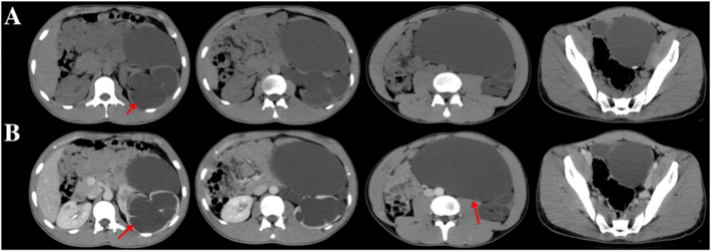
Case 5. CT scans obtained in a 20-year-old man with left congenital megaloureter. Nonenhanced scan obtained at the abdomen & pelvis shows huge fluid-filled loops (*red arrow*), and the normal bowel loops are compressed to the right side of the abdominal wall (a). The left renal pelvis and calyces show marked dilation, with parenchyma thinning (*red arrow*) on parenchymal phase enhancement (b), for unknown reason the images of prolonged phase were missing
